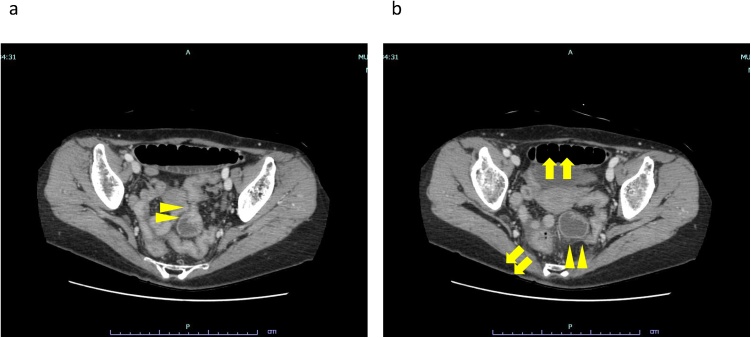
abdominal CT. a: A closed loop with the broad ligament of the uterus as the herniaorifice. b: A small bowel dilation in the pelvis (arrowheads). The uterus was slightly shifted ventrally and the recto-sigmoid shifted dorso-laterally (arrows).
